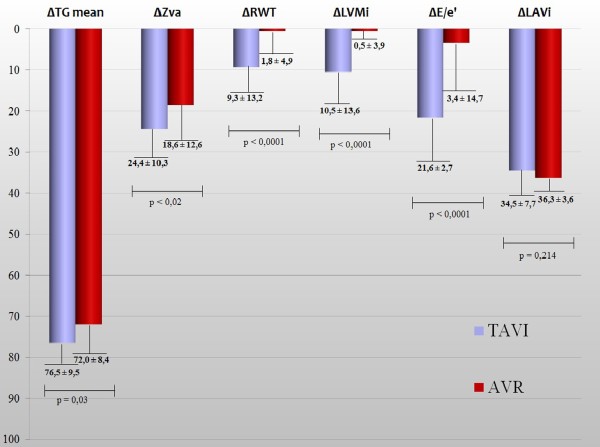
Comparison of per cent reduction of the main echo Doppler parameters in TAVI and AVR groups.
